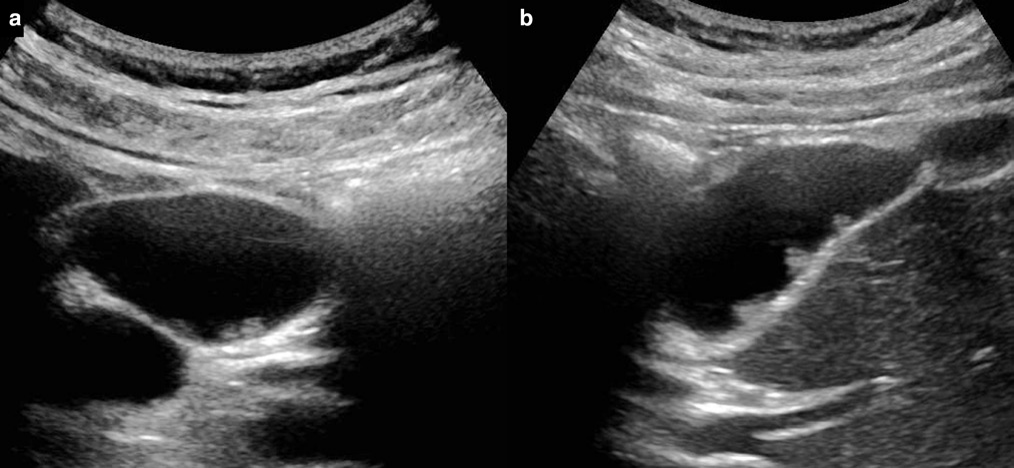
(a, b) Greyscale ultrasound images in transverse and longitudinal sections demonstrating multiple subcentimetre echogenic foci within the gallbladder arising from the posterior wall. Appearances are typical of small polyps (although difficult to differentiate true polyps from pseudopolyps on imaging). The polyps are non-mobile with no associated posterior acoustic shadowing helping to differentiate from stones.
